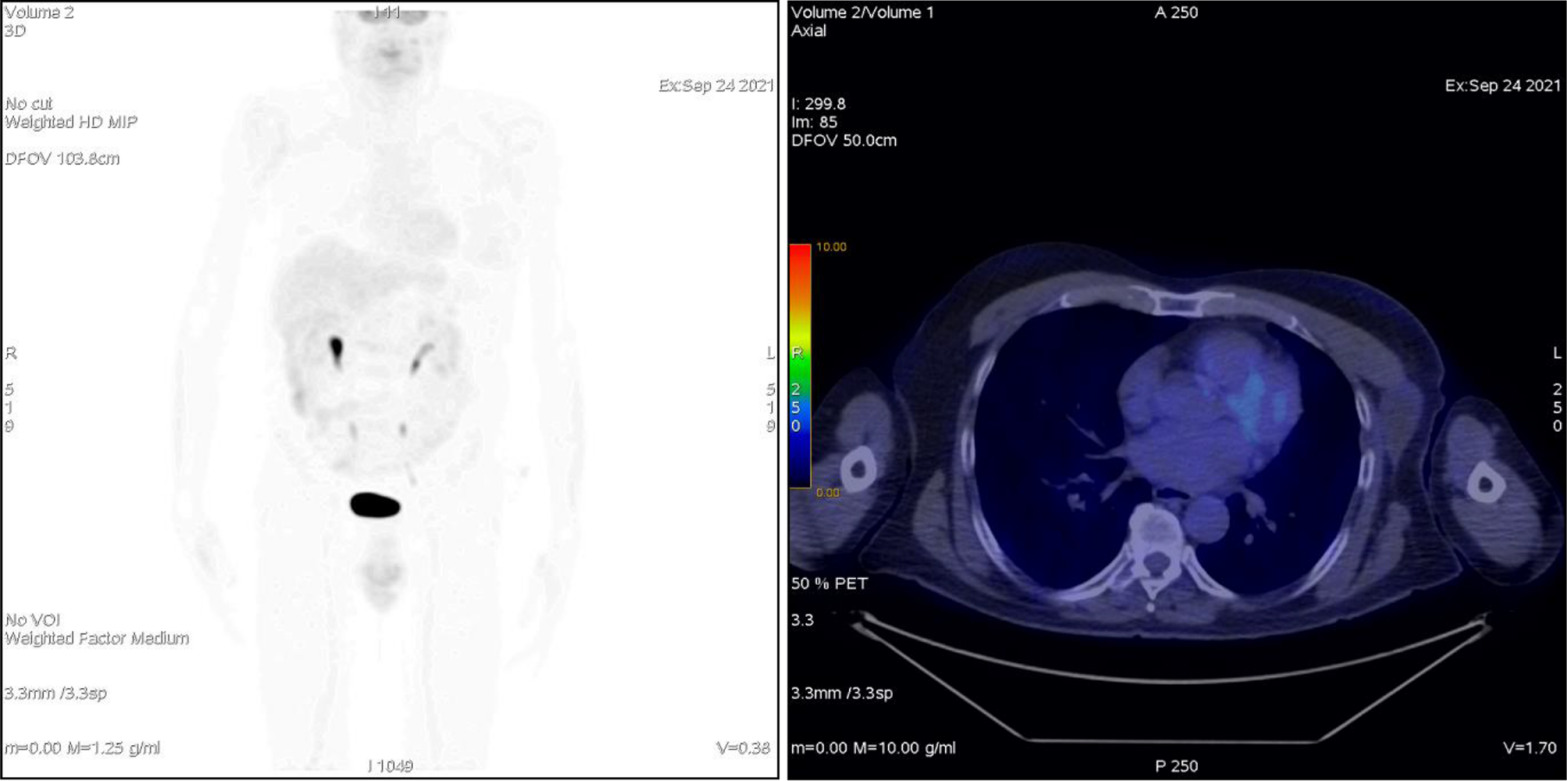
Patient ^18^F-FDG PET at 4.5 months after treatment.
